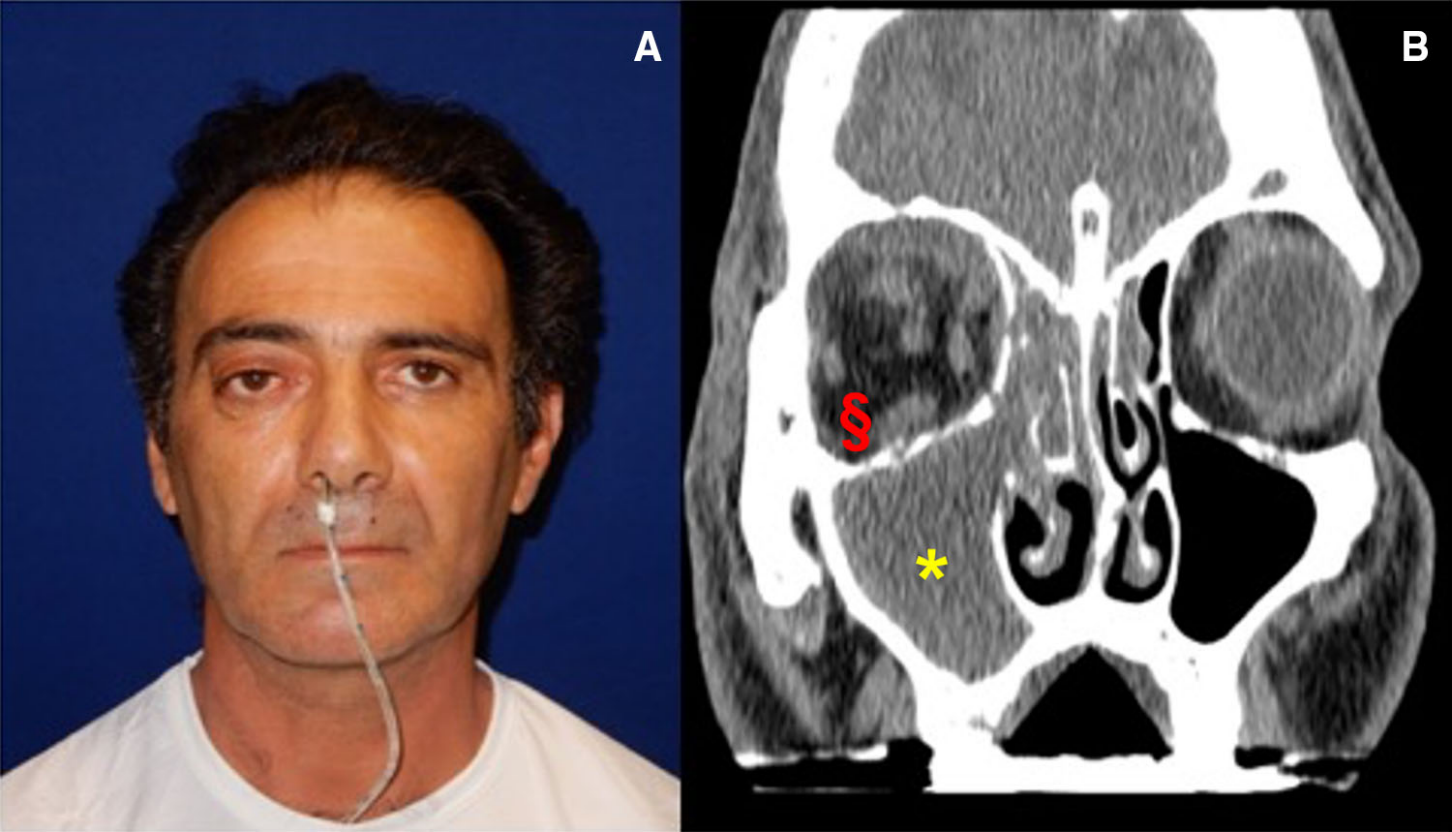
Case 1: Presurgical frontal photo: limitation movement of right eye not consensual with the left one (a) and CT Imaging in coronal section: maxillary sinus occupied by abscess (*), periorbital inflammatory degeneration of tissues (§) (b)
